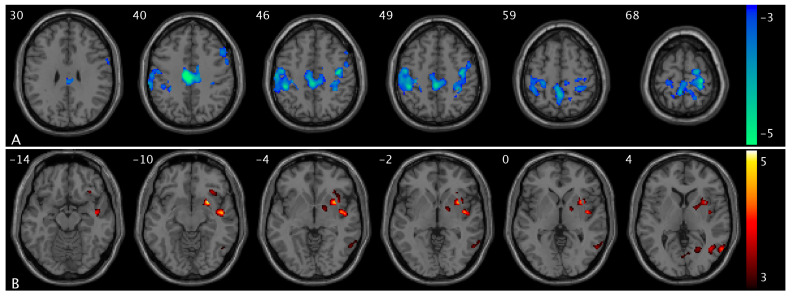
Statistical maps overlaid on T1-weighted images showing altered PCC FC in OAB participants compared to controls at different urge states. (A) Decreased PCC FC with the precentral, postcentral, and paracentral extending to inferior parietal cortex and middle cingulate regions and (B) increased PCC FC with the calcarine, insula, putamen, and pallidum regions in OAB participants compared to healthy controls in the low-urge state. The numbers in the top left corner of the MRI images indicate the z-coordinate (in mm) in the MNI space. The color bars represent t-values.
